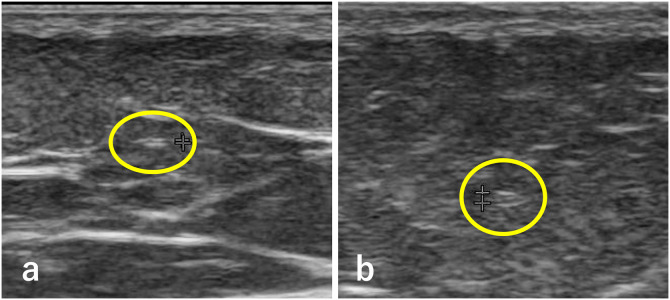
Lymphatic ultrasound findings. Yellow circles indicate the lymphatic vessels. (a) Normal lymphatic vessel without dilation, appearing as a high-echo structure resembling an equal sign. (b) Dilated lymphatic vessel due to lymphatic stasis.
